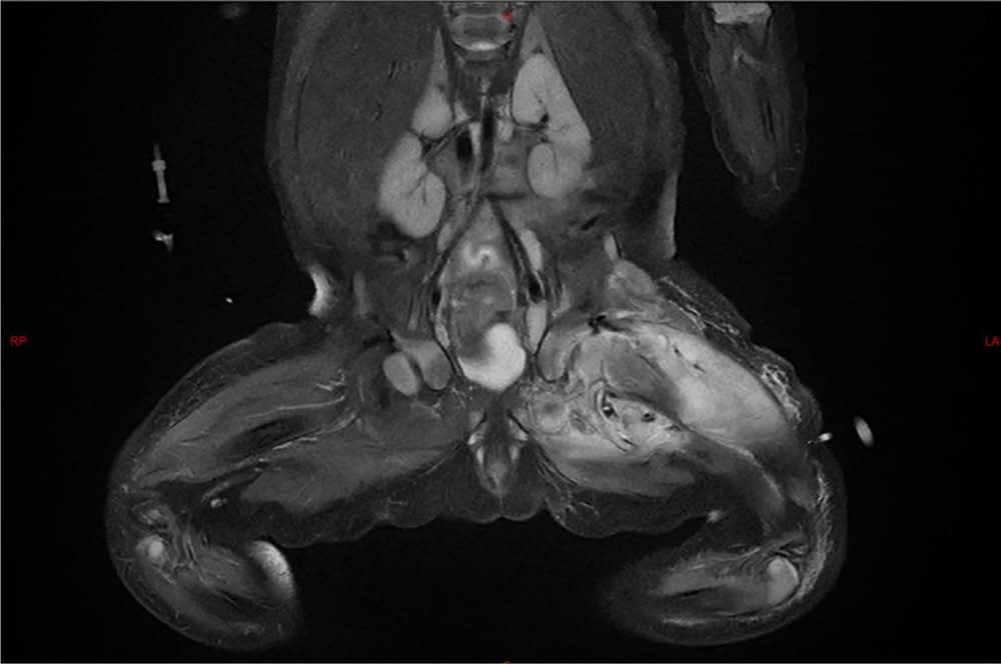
MRI of both hips in a 24-day-old neonate (Case 1). Left thigh abscess formation with infection of the left femoral head and hip joint. MRI = magnetic resonance imaging.
